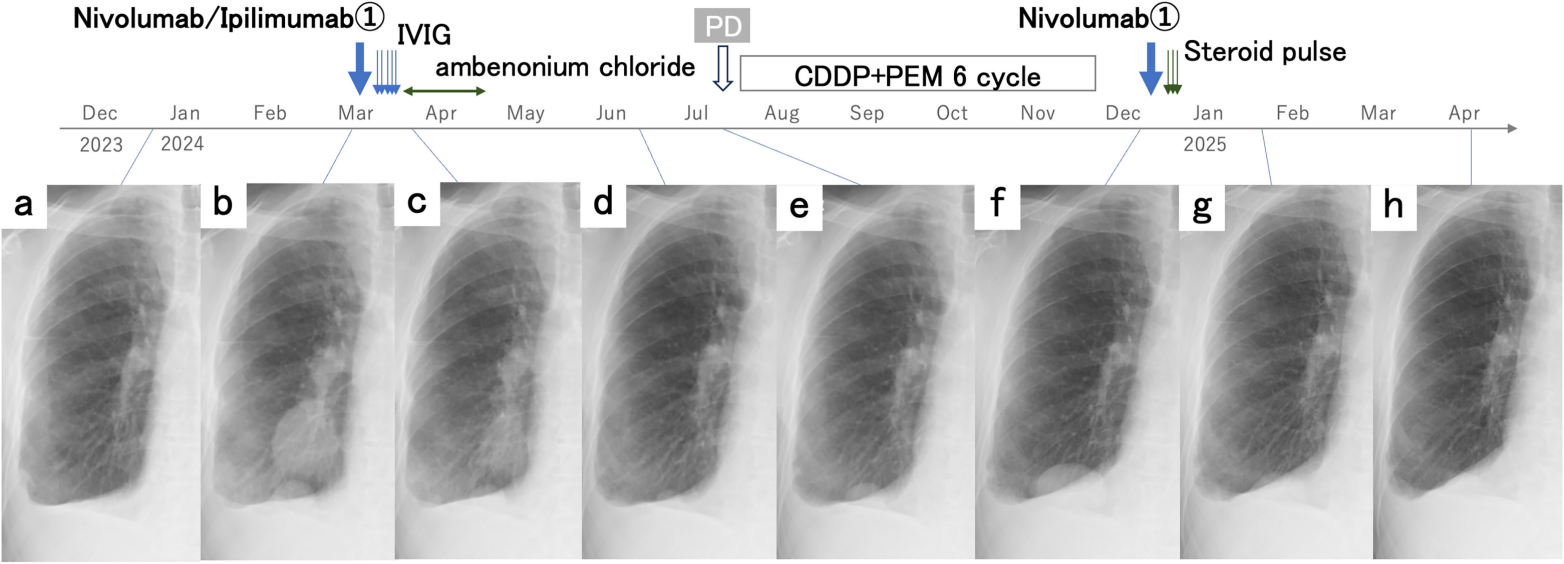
Treatment timeline and serial chest radiographs. (a) Seventy‐seven days before nivolumab plus ipilimumab administration. (b) Three days before administration, showing rapid tumour growth over approximately 2 months. (c) Fourteen days post‐administration, showing tumour shrinkage. During this period, prophylactic treatment for myasthenia gravis flare (intravenous immunoglobulin [IVIG] and oral ambenonium chloride) was administered. (d) On Day 101 post‐administration, a partial response (PR) was maintained; however, by Day 130, enlargement of the basal lung mass was observed, and progressive disease (PD) was diagnosed (e). Cisplatin (CDDP) and pemetrexed (PEM) were subsequently administered for six cycles, resulting in stable disease as the best‐observed response; however, the mass gradually increased. (f) One day before nivolumab monotherapy. Steroid pulse therapy was initiated in response to elevated CK levels on Day 7 after administration. (g) On Day 45, post‐nivolumab monotherapy, shrinkage of the basal lung mass was observed, and PR was sustained through Day 114.
